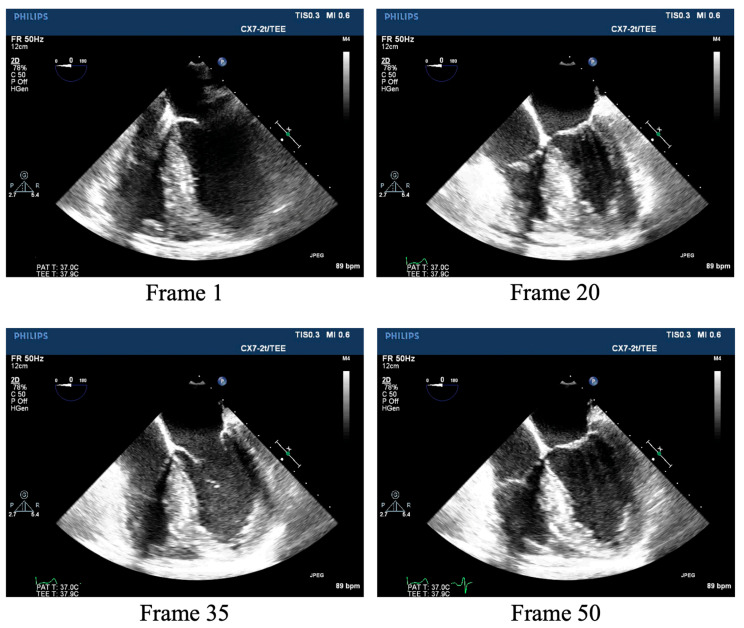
The 1st, 20th, 35th, and 50th TEE A4CV frames of a TEE video of the same patient. TEE: transesophageal echocardiography; A4CV: apical four-chamber view.
